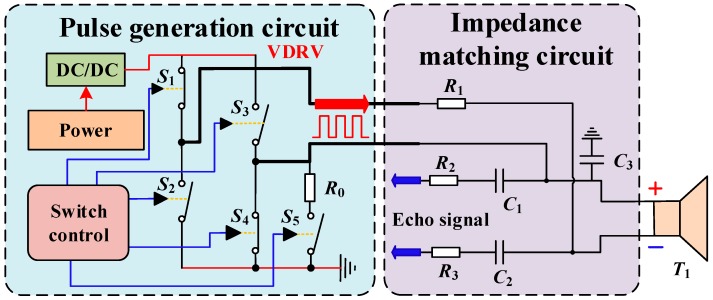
Intrinsically safe ultrasonic driving circuit.
